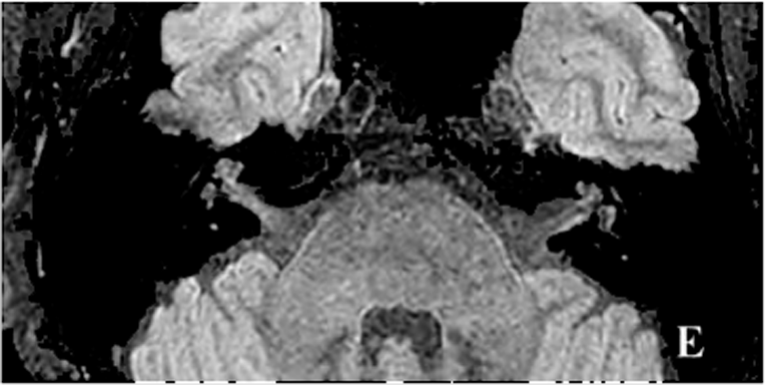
In July 2021, under gadolinium angiography in the 3D-T2-FLAIR sequence, left-sided EH improved (E).
